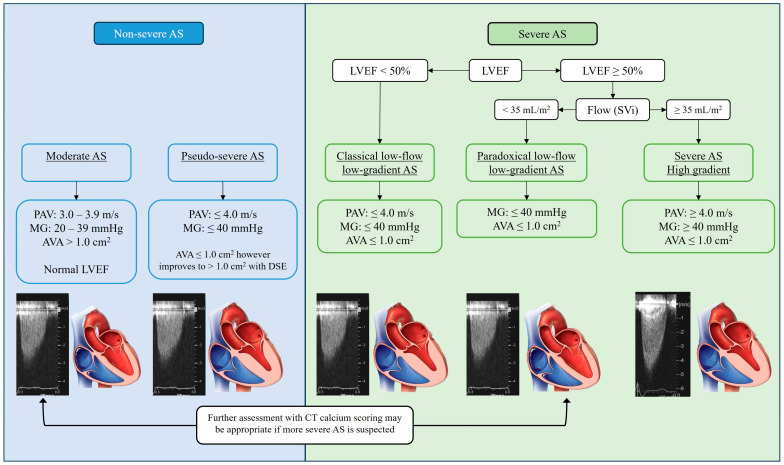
Flow-chart of classification of aortic stenosis (AS) grading based on echocardiographic measurements. Figure has been based off published work with permission from [24]. Copyright 2017 Elsevier. Abbreviations; Aortic stenosis (AS); aortic valve area (AVA); mean gradient across aortic valve (MG); peak velocity across aortic valve (PAV); left ventricular (LV); left ventricular ejection fraction (LVEF); dobutamine stress echocardiography (DSE); stroke volume index (SVi); computed tomography (CT).
